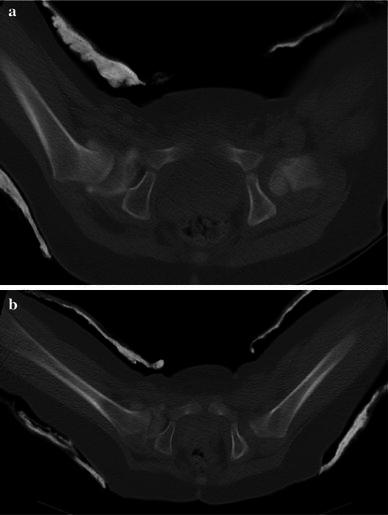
a Postreduction single slice CT image with hips in neutral position compared with the hips in a more abducted position b in the same patient following change of spica cast
